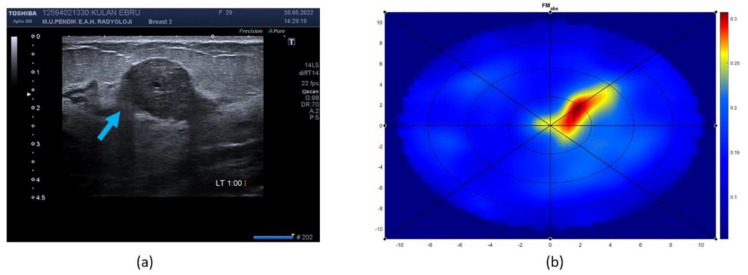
Imaging performance of ultrasound and SAFE: (a) ultrasound scan axial showing the presence of benign lesion in small breast of unknown density, and (b) SAFE scan confirming the presence of benign lesion (high contrast region), inside the scanning domain (blue circle), at the same location as reported by ultrasound.
